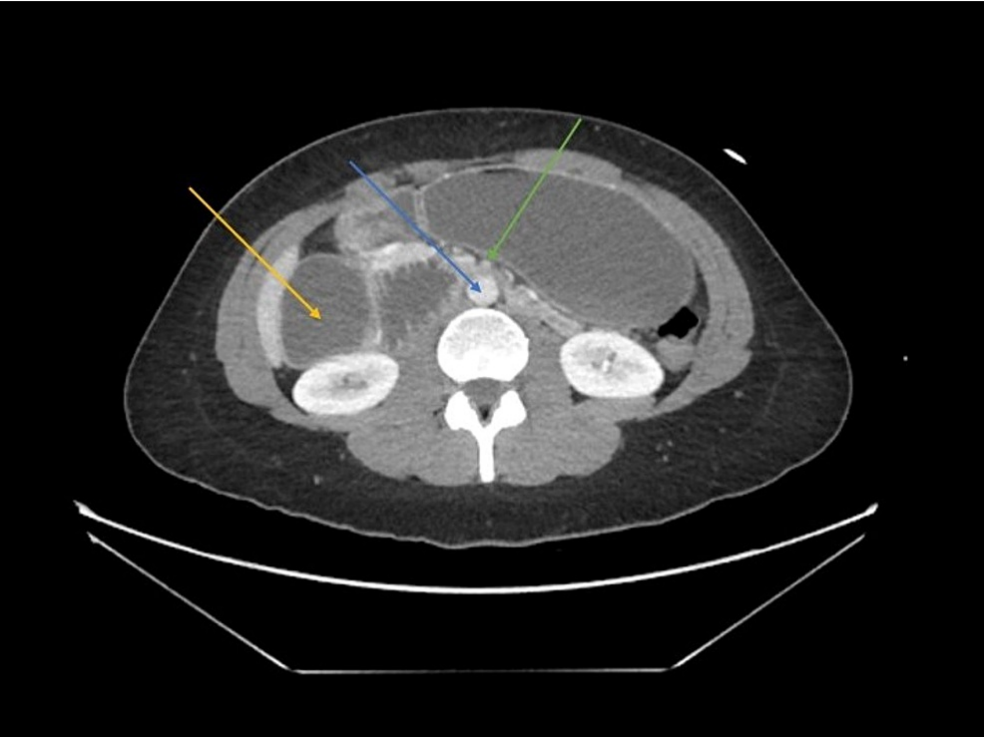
CT scan of the patient The SMA (right, green arrow) can be visualized anterior to the aorta (middle, blue arrow) with dilated proximal duodenum (left, yellow arrow) CT: computed tomography; SMA: superior mesenteric artery
